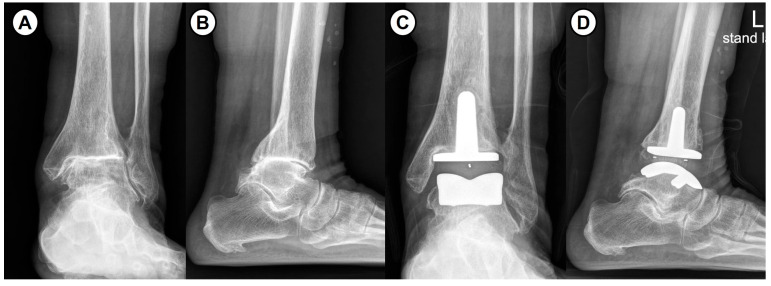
(A,B) Preoperative and (C,D) postoperative radiographs in a 68-year-old female with total ankle arthroplasty for end-stage rheumatoid arthritis.
